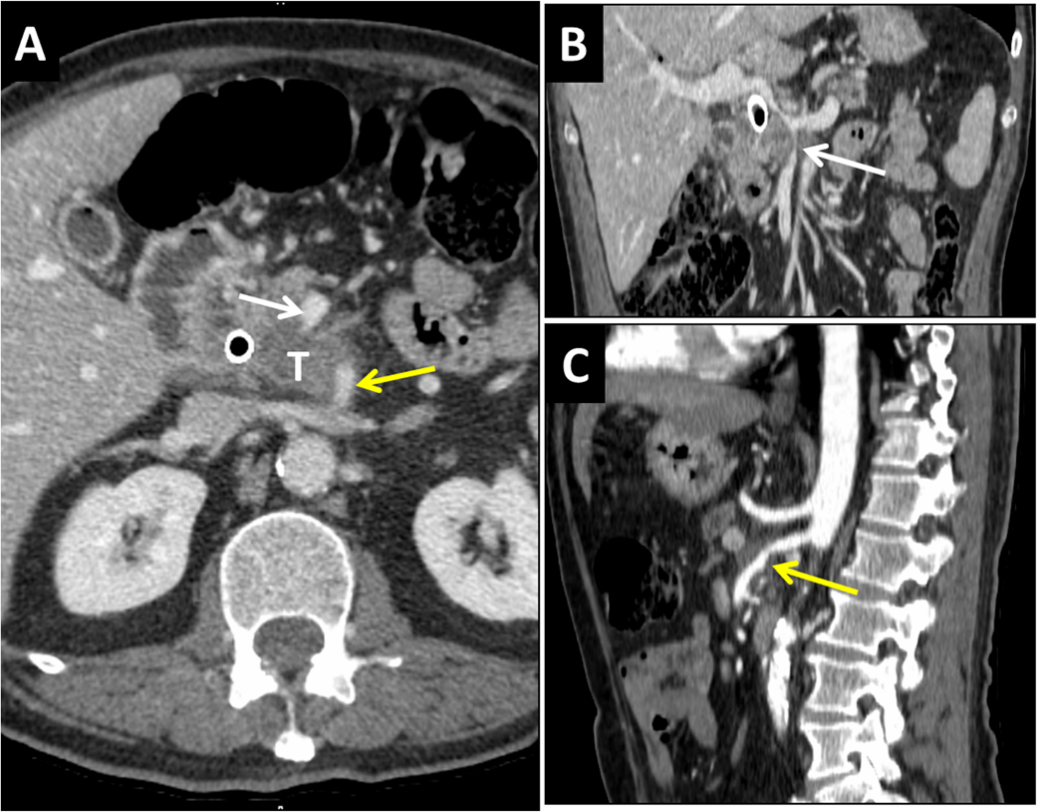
Computed tomography (CT) scan of patient number 1 showing a tumor of the head of the pancreas (T) with infiltration of both the superior mesenteric vein (SMV) (white arrow) and the superior mesenteric artery (SMA) (yellow arrow). (A) Axial view; (B) frontal view; (C) sagittal view.
